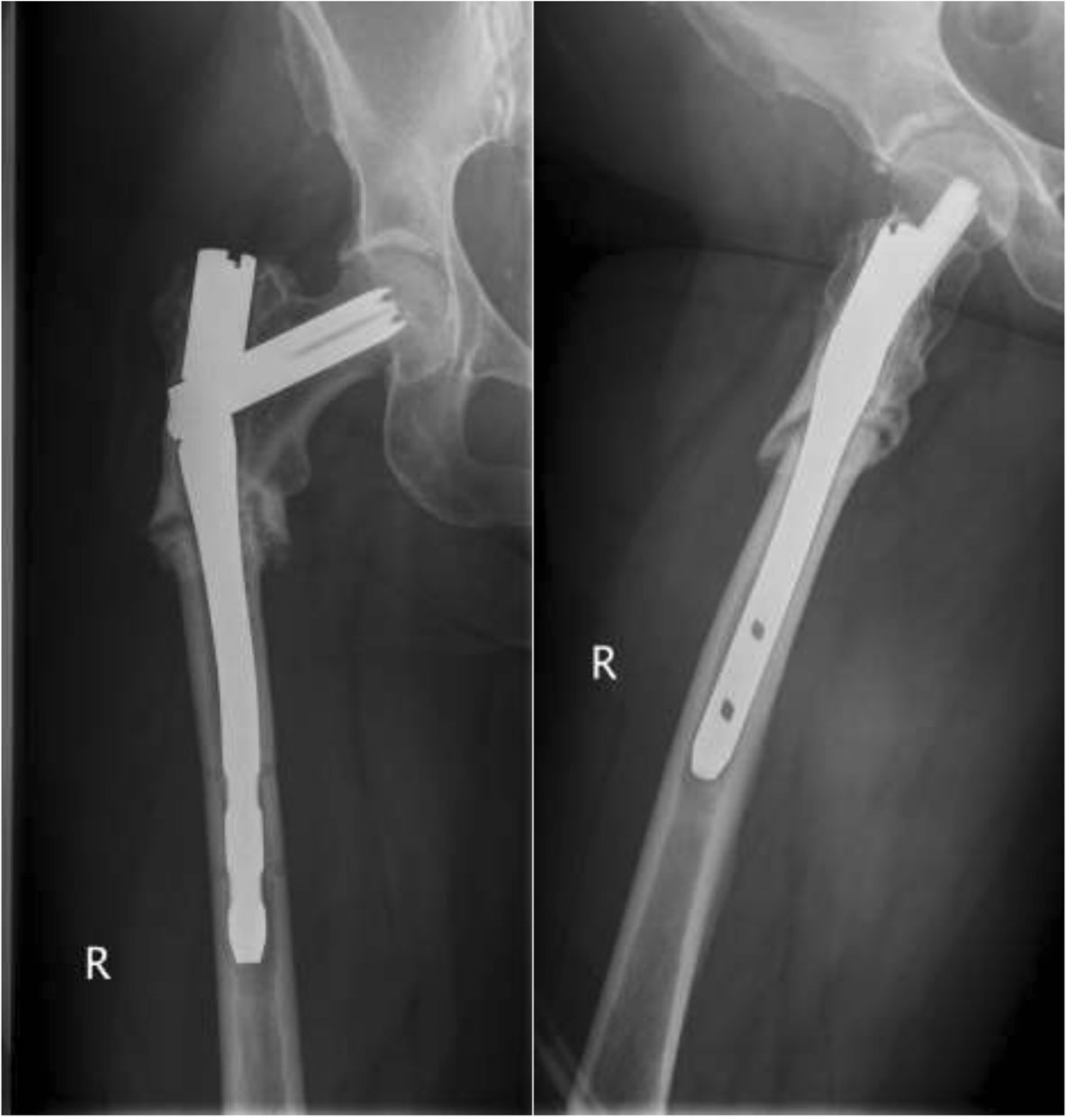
AP and lateral radiographs of a 56-year-old female patient with a hypertrophic nonunion after nailing with an implant failure and varus nonunion of the femur with a CCD of 109
